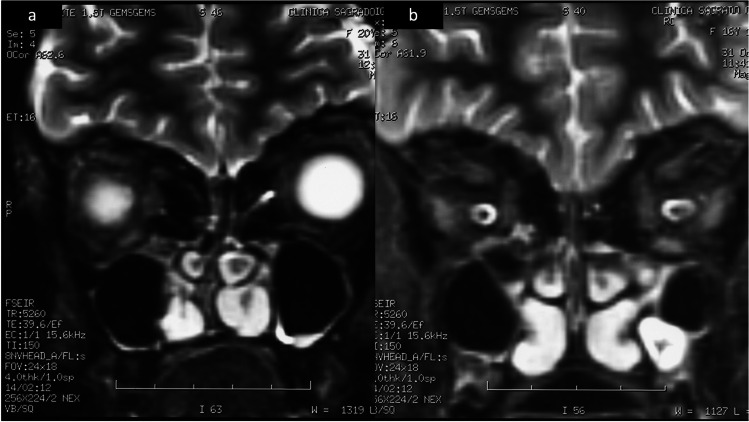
Brain MRI revealed aplasia in the olfactory bulbs and tract in both patients (case 1 (a) and 2 (b))
